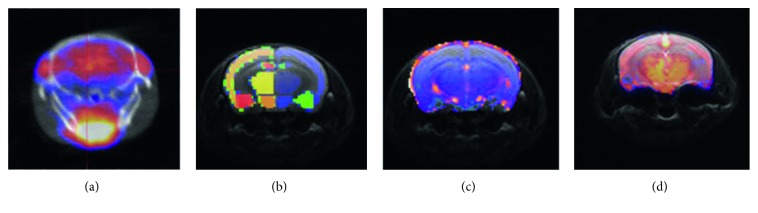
[^18^F]FDG-PET-CT coregistered to MRI images (a). Altas coregistered T2w turboRARE (b). ADC map coregistered to T2w turboRARE (c). Perfusion map coregistered to T2w turboRARE (d) (all images are from individual studies).
